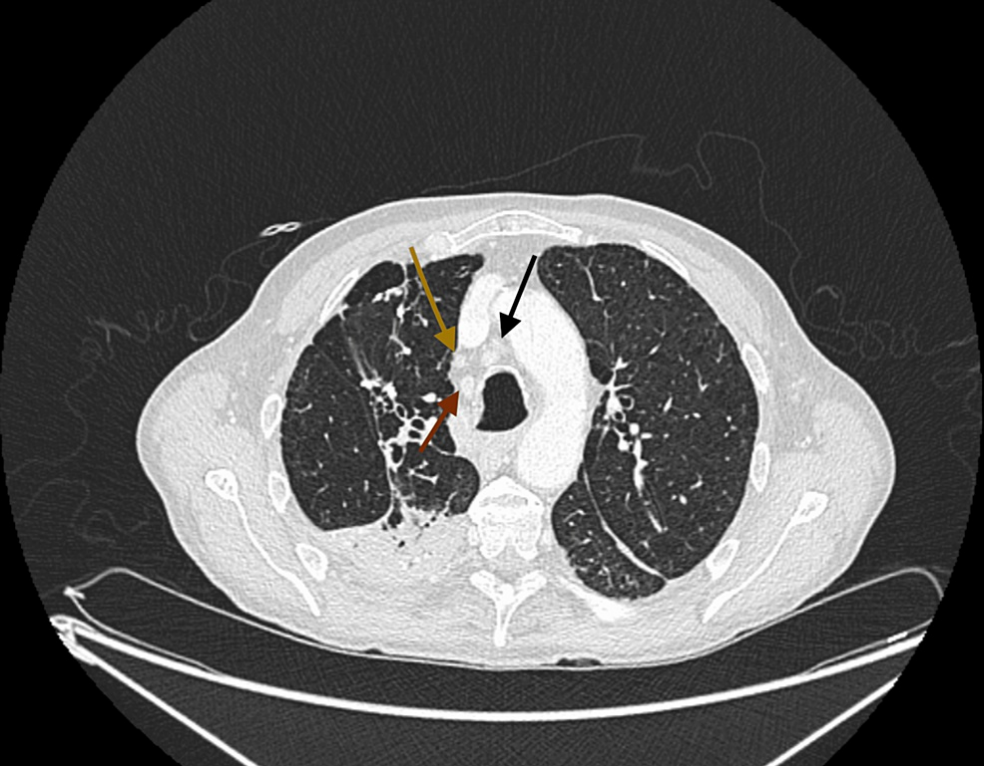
The CT thoracic tomography revealed multiple mediastinal lymphadenopathies red arrow: inferior pretracheal node on the right measuring 17 mm in short axis; yellow and black arrows: infracarinal node measuring 16 mm, and right hilar node measuring 11 mm
